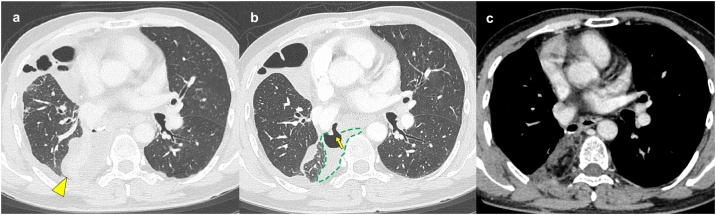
Computed tomography showing the condition of the stump of the right lower lobe: only atelectasis of the middle lobe on day 6 (arrowhead) (a), and bronchopleural fistula (arrow) connecting to the limited pleural space (dashed line) was confirmed on day 12 (b). Bronchopleural fistula and pleural space were closed with the latissimus dorsi muscle without recurrence 9 months after closure (c).
